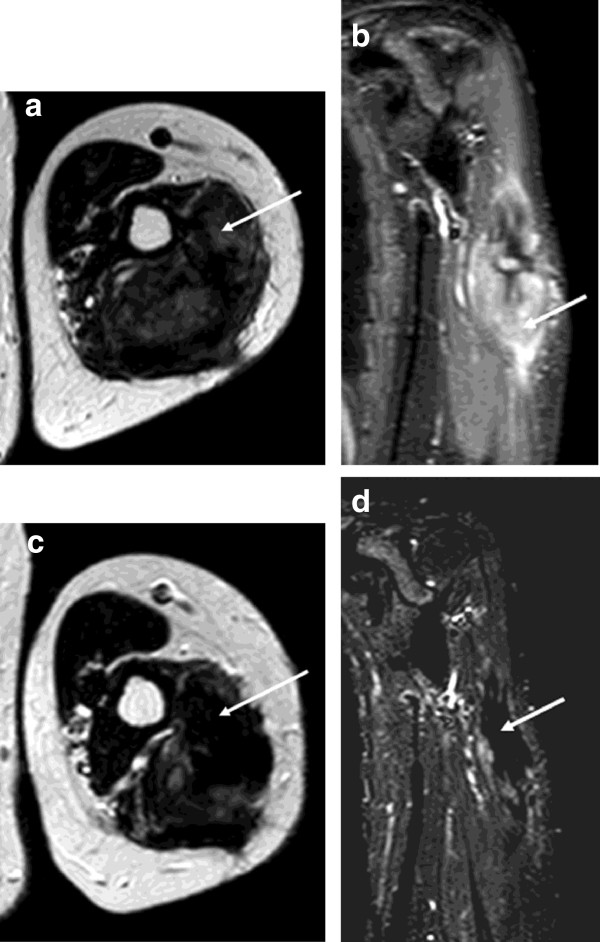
Axial T2 weighted and coronal STIR MRI images of the proximal left arm at baseline (a and b) and following 11 months of pazopanib (c and d). A large focus of fibromatosis expands the triceps muscle and following 11 months of treatment reduced in size from 10.2 cm in maximum craniocaudal dimension to 8.0 cm. Predominantly intermediate/high signal tissue (a and b, arrows) showed a marked reduction in signal (c and d, arrows) indicating diminished cellularity.
